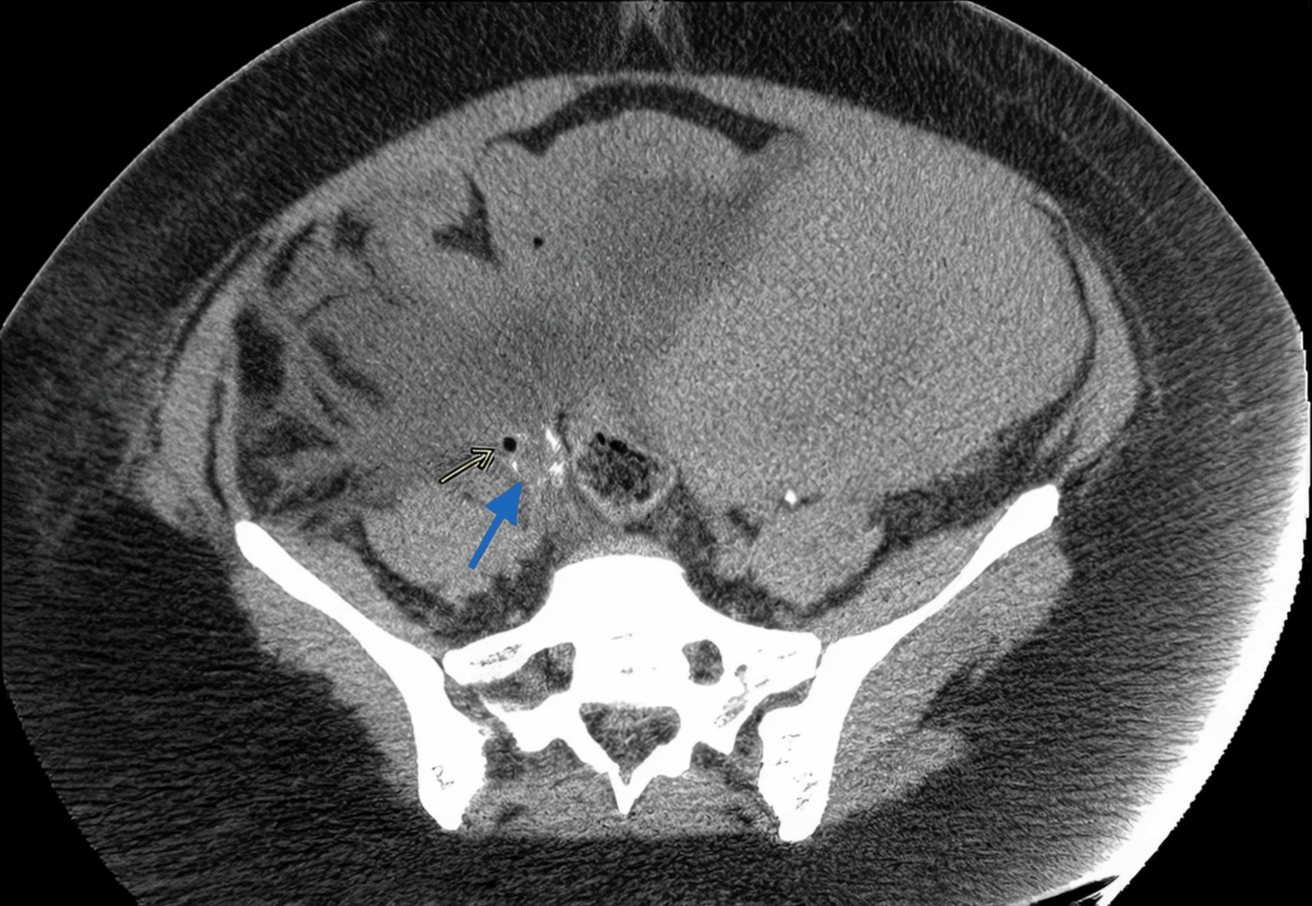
CT post-contrast delayed phase axial image: confirming the extravasation of contrast from the distal segment of the right ureter just distal to the right iliac vessels bifurcation (arrow). Air foci near the lumen of the right ureter confirm the site of the leak.
